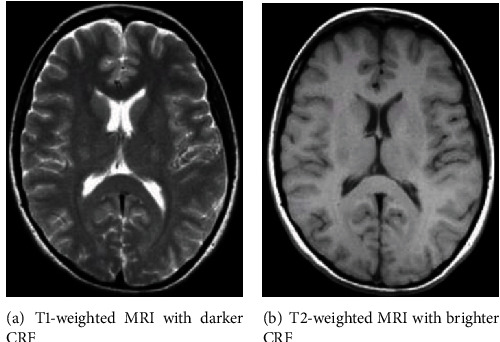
Samples of T1-weighted and T2-weighted brain MRI with different intensity values of cerebrospinal fluid (CRF).
